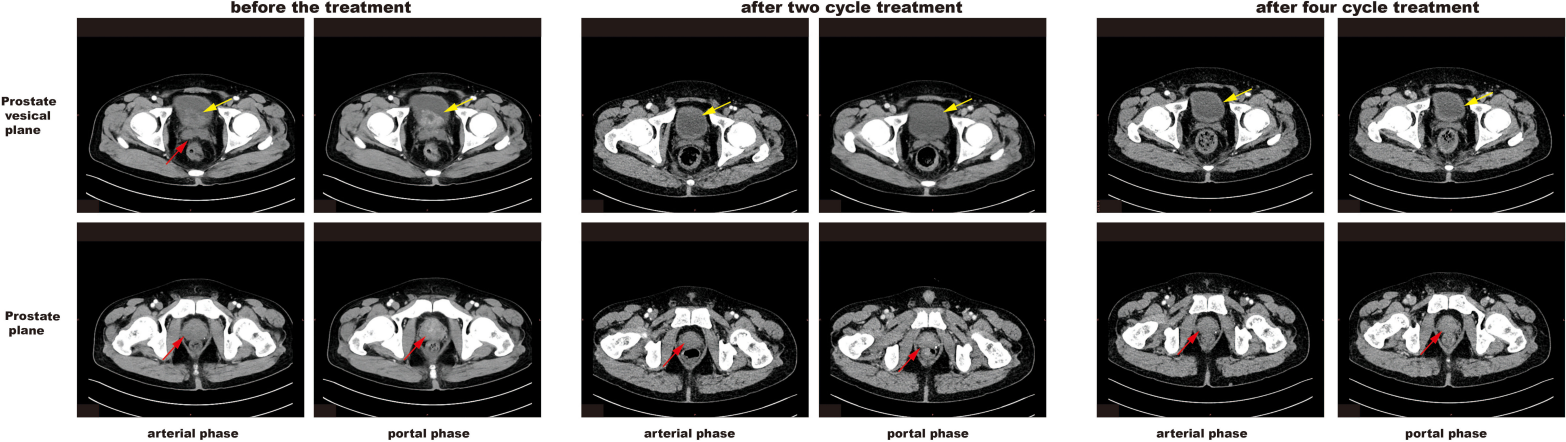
Representative CT images of the primary PCa site before and after treatment. Yellow arrows and red arrows indicate the gradually decreased prostate at the prostate vesical plane and prostate plane, respectively. After four cycles of treatment, the CT images revealed no appreciable abnormal contrast-enhanced lesions.
